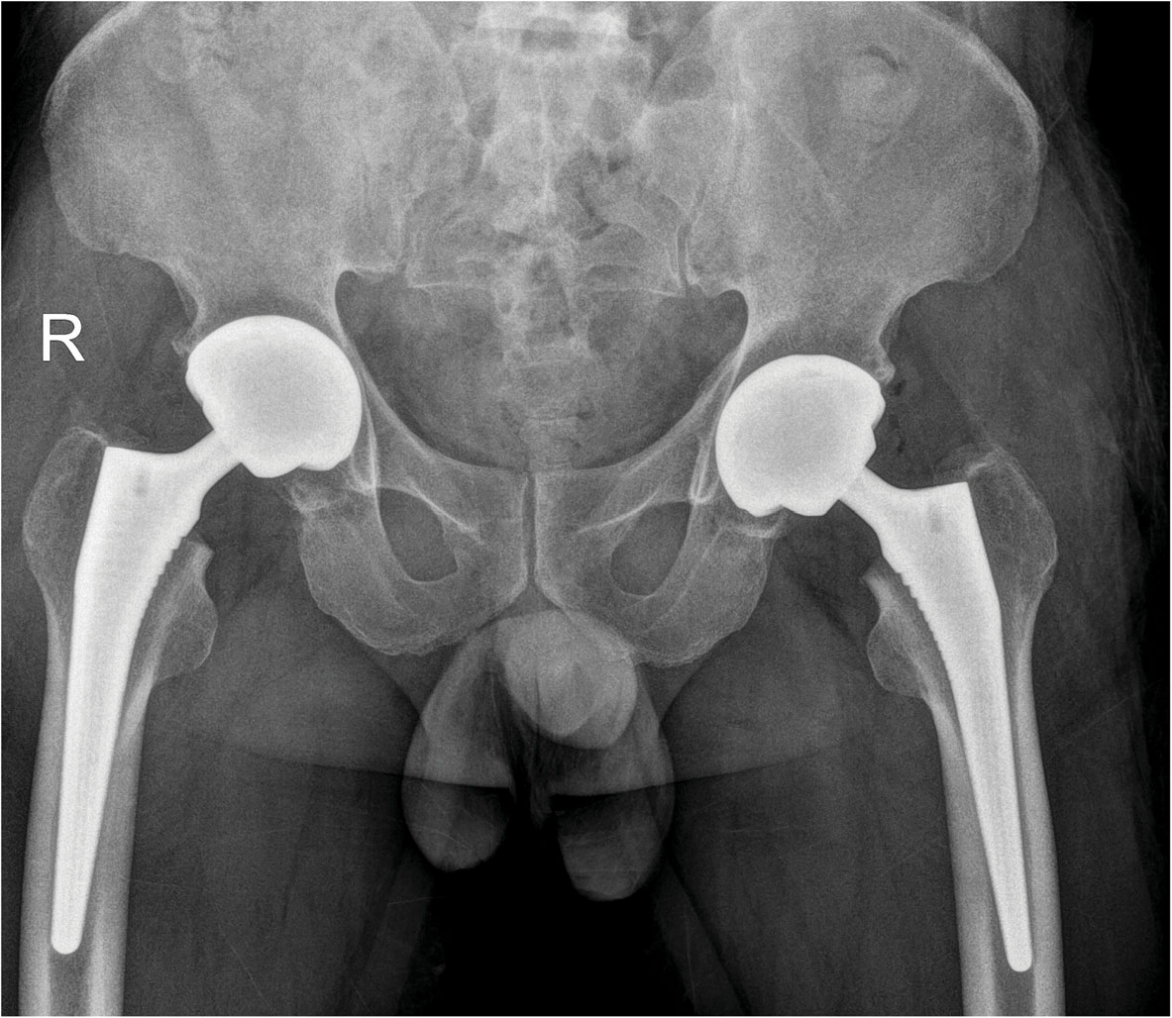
Plain radiograph after THA
